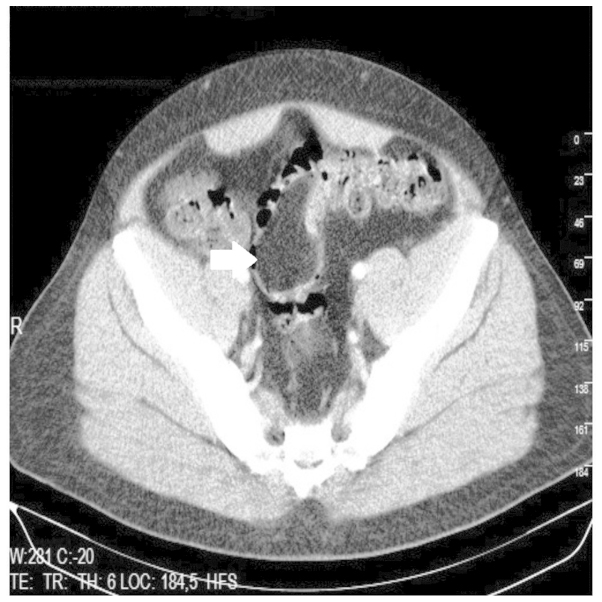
Computed tomography image of the mass (arrow).
